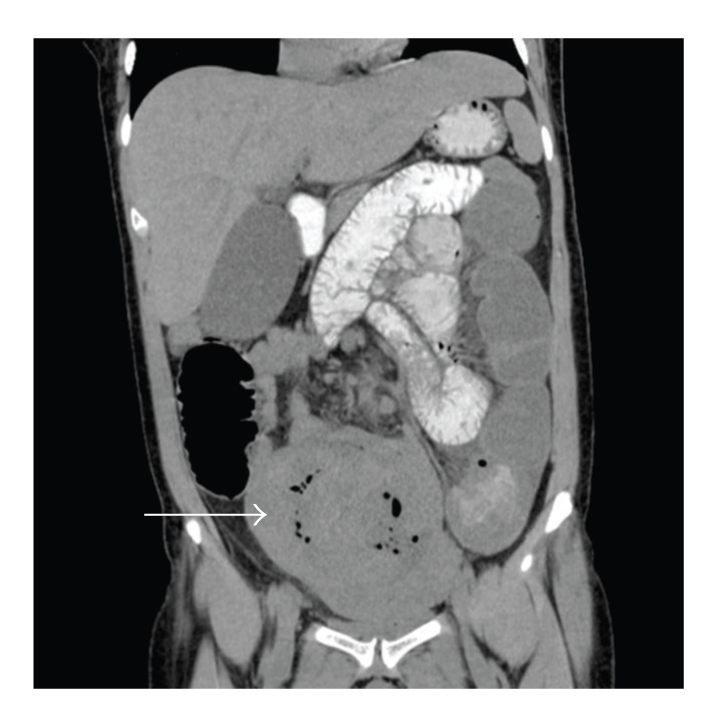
CT scan of the patient's abdomen on postpartum day 27 demonstrating the uterus with the retained placenta was demonstrated (arrow). The presence of dilated, fluid filled loops of small bowel with collapse of the large bowel confirmed the diagnosis of a mechanical small bowel obstruction. A possible adhesion was noted between the uterine fundus and the bowel (not shown here).
